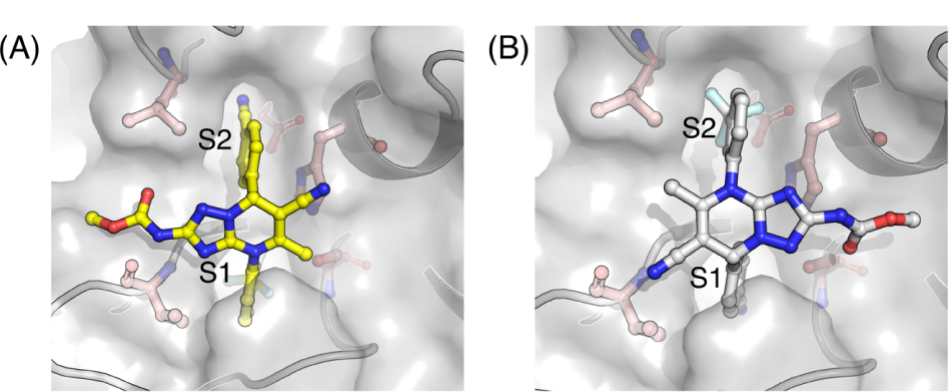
Compound 2 bound to HNE in the (A) X-ray pose and (B)
